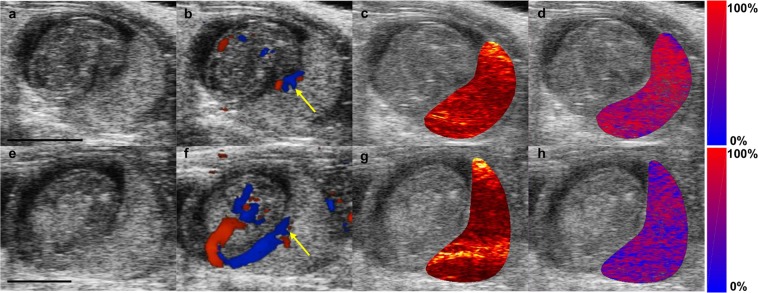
B-mode US images of the placental environment, (a,e), in NP, (a–d), and the RUPP, (e–h), at GD 16. (b,f) show the Color Doppler ultrasound of blood flow the umbilical cord. The yellow arrow indicates the origin of the umbilical cord on the placenta which was used as a landmark for determining the imaging field. (c,g), show the accumulation of the photoacoustic signal intensity at 808 nm segmented to the placental. At the isosbestic point, the photoacoustic signal is generated equally by both Hb and Hb_O2_. The custom colormap of oxygen saturation, segmented to the placenta and superimposed on the ultrasound image, is shown in (d,h). Red denotes completely oxygenated blood while blue denotes completely deoxygenated blood. Scale bars are 3 mm.
